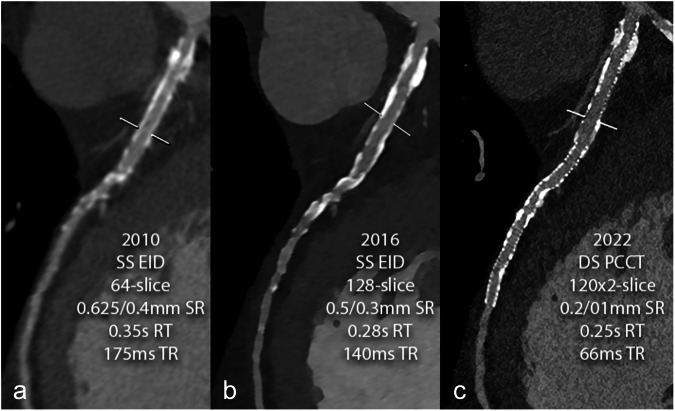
Clinical evolution of cardiac CT angiography (CCTA) performance across 15 years in the assessment of stent patency. The same approximately 70-year-old patient with known coronary artery disease, who was followed for a long time using CCTA for multiple coronary stents; the image focuses on the left anterior coronary artery with several stents from the ostium; it is evident that, along the follow-up, more stents have been positioned more and more distally along the vessel. a CCTA scan performed in 2010 with a single-source (SS) 64-slice CT with 0.625 mm slice thickness and 175 ms temporal resolution, reconstructed with a curved multiplanar reconstruction (MPR). b The same curved MPR is derived from a CT scan performed in 2016 with a SS 128-slice CT with 0.5 mm slice thickness and 140 ms temporal resolution. c The same curved MPR is derived from a dual source (DS) PCCT scan performed in 2022 on a first-generation DS 120 × 0.2 mm × 2, with 0.2-mm slice thickness and 66 ms temporal resolution. The progression in image sharpness is evident, as well as the reduction of the impact of motion artifacts and blooming artifacts related to metal stent structs and calcifications. CCTA, Cardiac CT angiography; CAD, Coronary artery disease; DS, Dual source; EID, Energy-integrating detector; MPR, Multiplanar reconstruction; PCD, Photon-counting detector; PCCT, Photon-counting CT; RT, Rotation time of the gantry; SR, Spatial resolution; SS, Single source; TR, Temporal resolution (in hardware)
